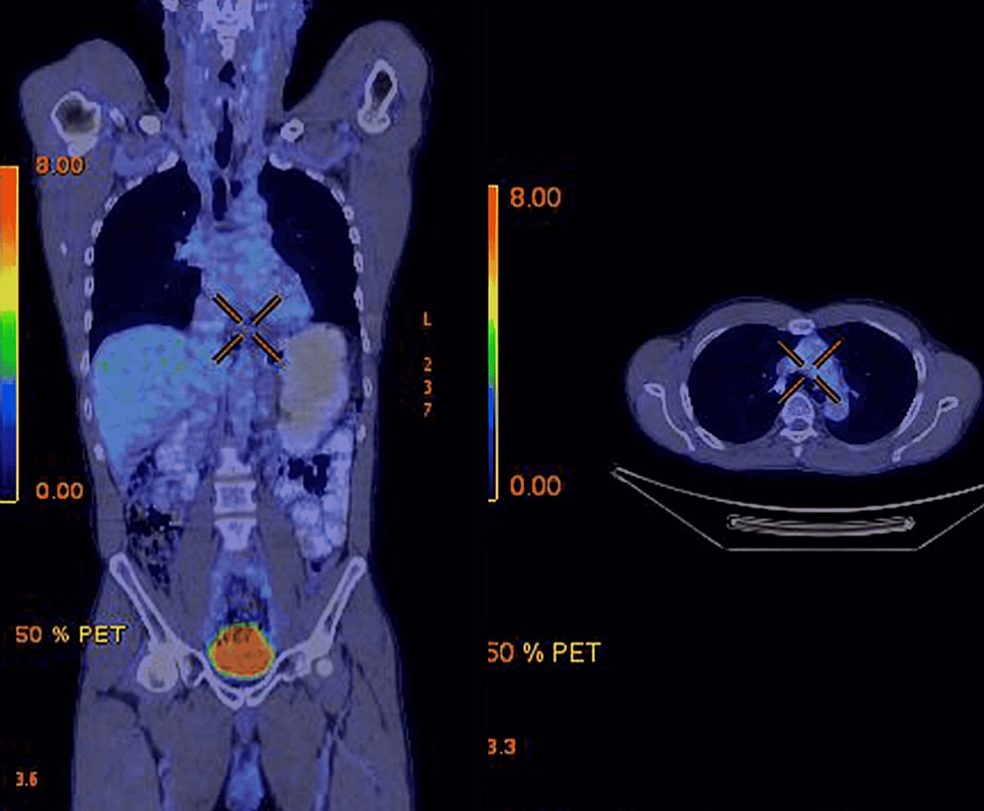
Post treatment PET-CT scan showing complete metabolic response
